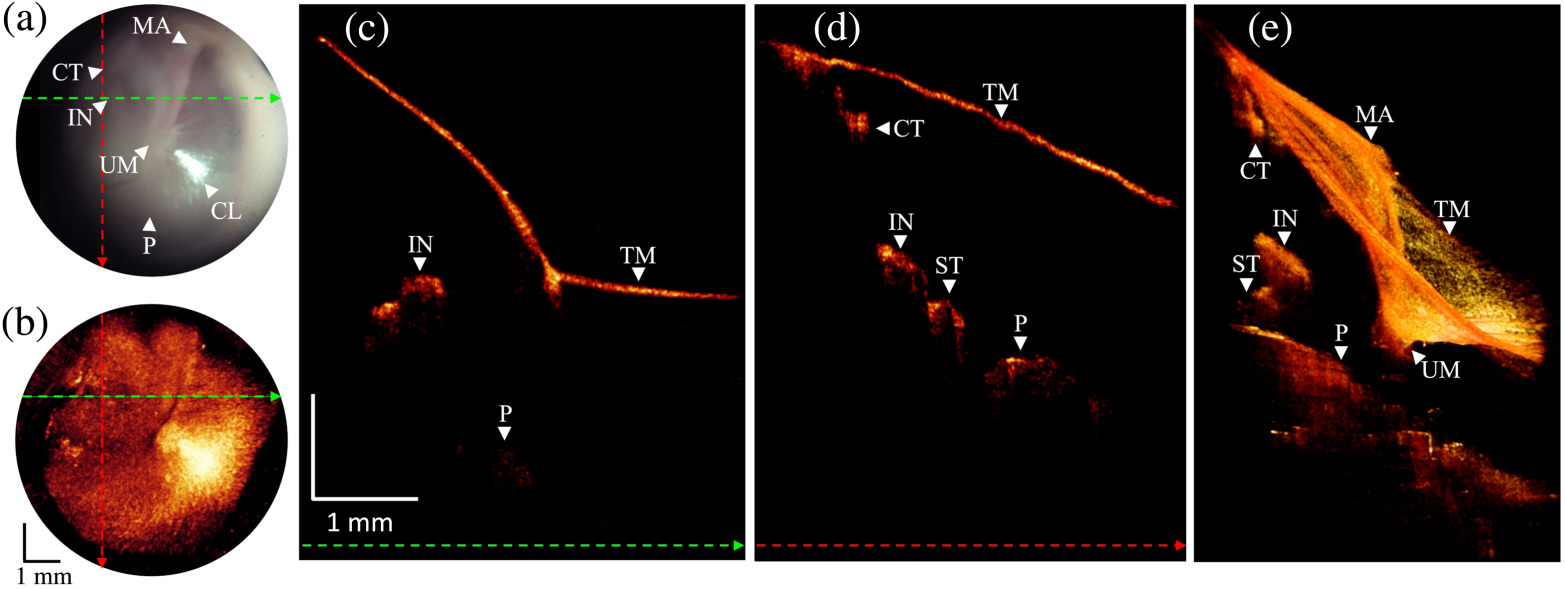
Otoscope and OCT image acquired from normal ear. Abbreviations: MA, malleus; CT, chorda tympani; TM, tympanic membrane; IN, incus; UM, umbo; CL, cone of light; P, promontory. (a) A snapshot of the otoscopic video camera. Dashed colored lines indicate the positions where the linear B-scans are shown in panels (c) and (d). (b) *En face* image generated by SVP from an OCT volume. Dashed colored lines indicate the positions where the linear B-scans are shown in panels (c) and (d). (c) B-scan extracted along the green-colored dashed line from the OCT volume. (d) B-scan extracted along the red-colored dashed line from the OCT volume. (e) 3D volume rendering.
